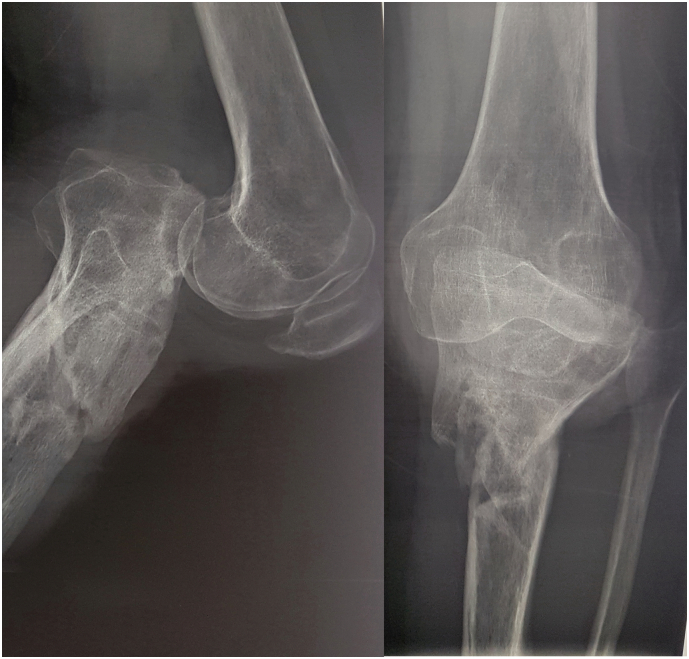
Preoperative knee radiographs showing a posterior knee dislocation with a bone callus on the proximal third of the tibial shaft.
